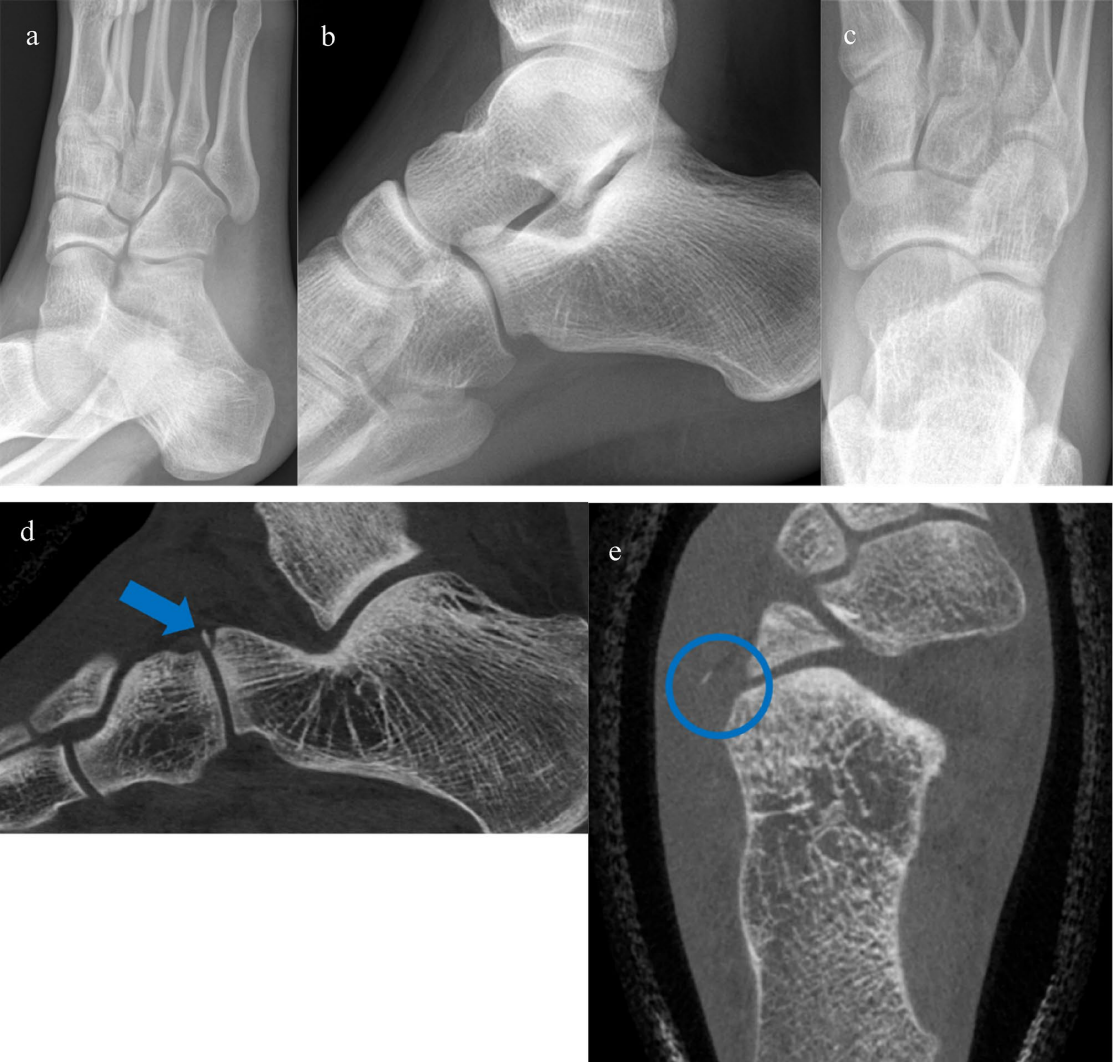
A 17-year-old patient with persistent pain after ankle traumatism with no visible fracture on XR a oblique, b lateral and c antero-posterior views. CBCT of the same patient d lateral and e axial views. The arrow points to an anterior calcaneal process fracture. The circle shows a dorsal calcaneocuboid fracture
